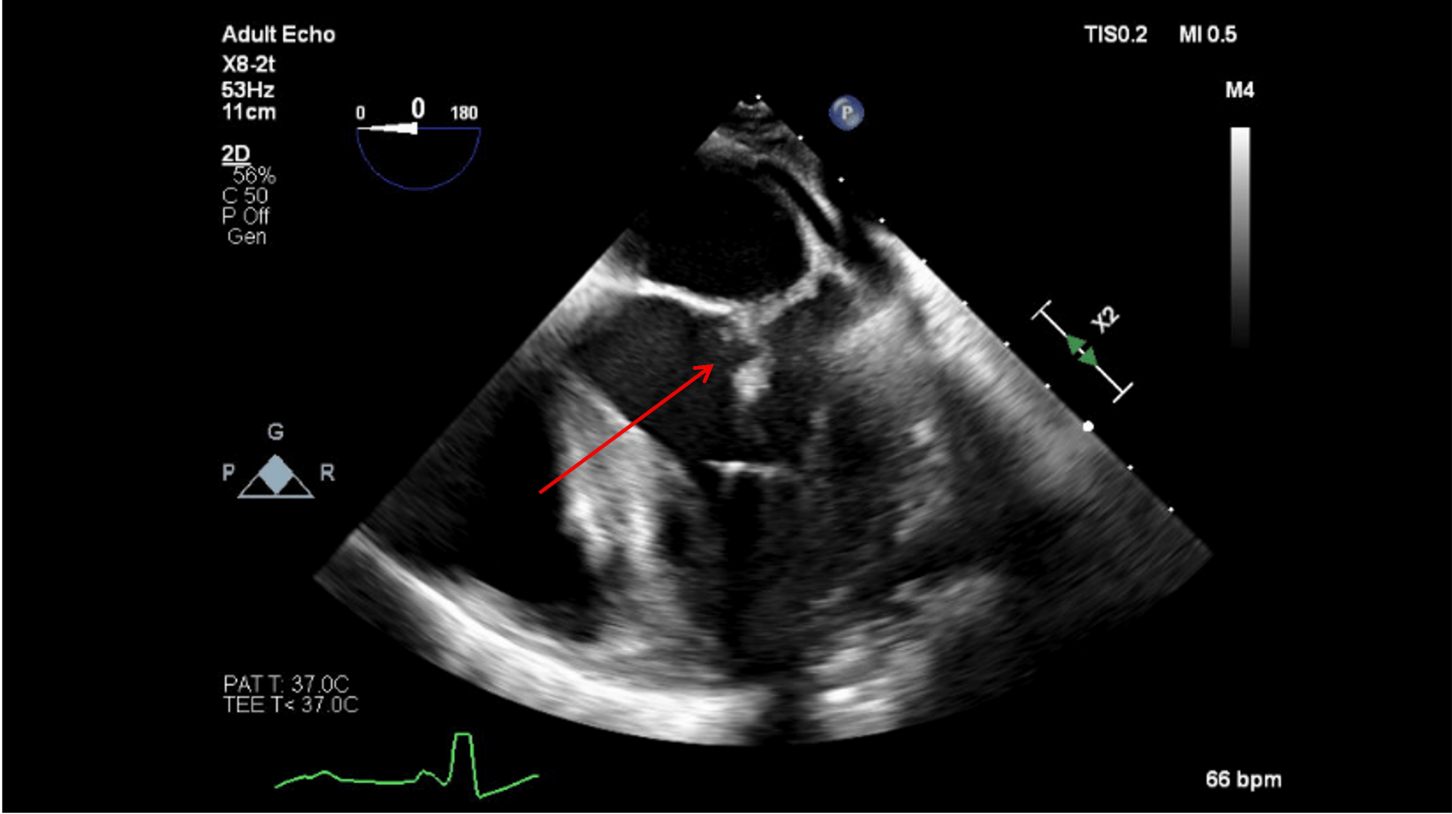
Mitral valve vegetation still visible on day four via transesophageal echocardiogram (red arrow).
